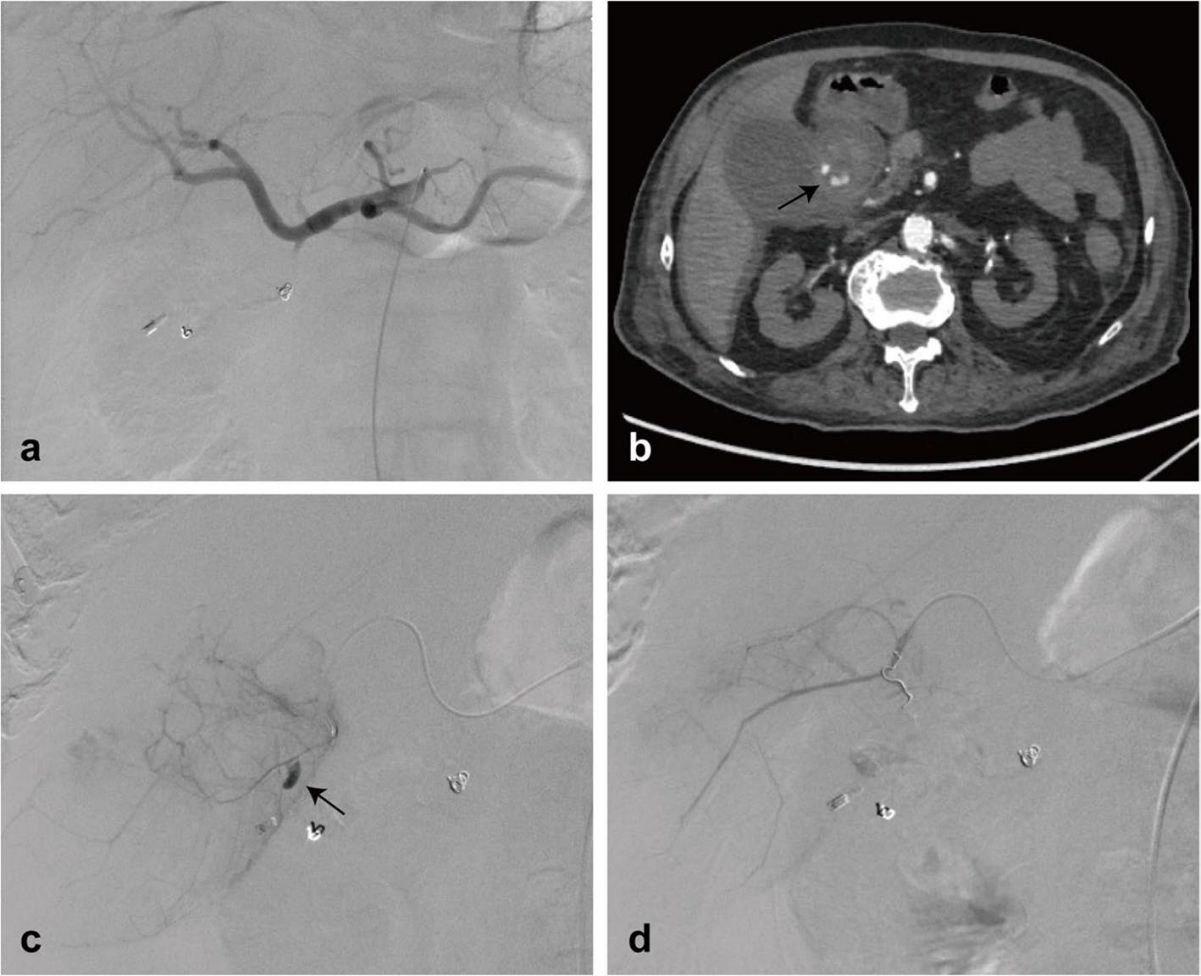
Case 1. a Angiography after empirical gastroduodenal artery embolization shows no positive sign. b Contrast-enhanced computed tomography demonstrates contrast extravasation near the gallbladder (arrow). c Super-selective angiography of the cystic artery demonstrates contrast extravasation (arrow). d Post-embolization angiography confirms successful occlusion of the bleeding branches of the cystic artery with gelatin sponge particles and coils
